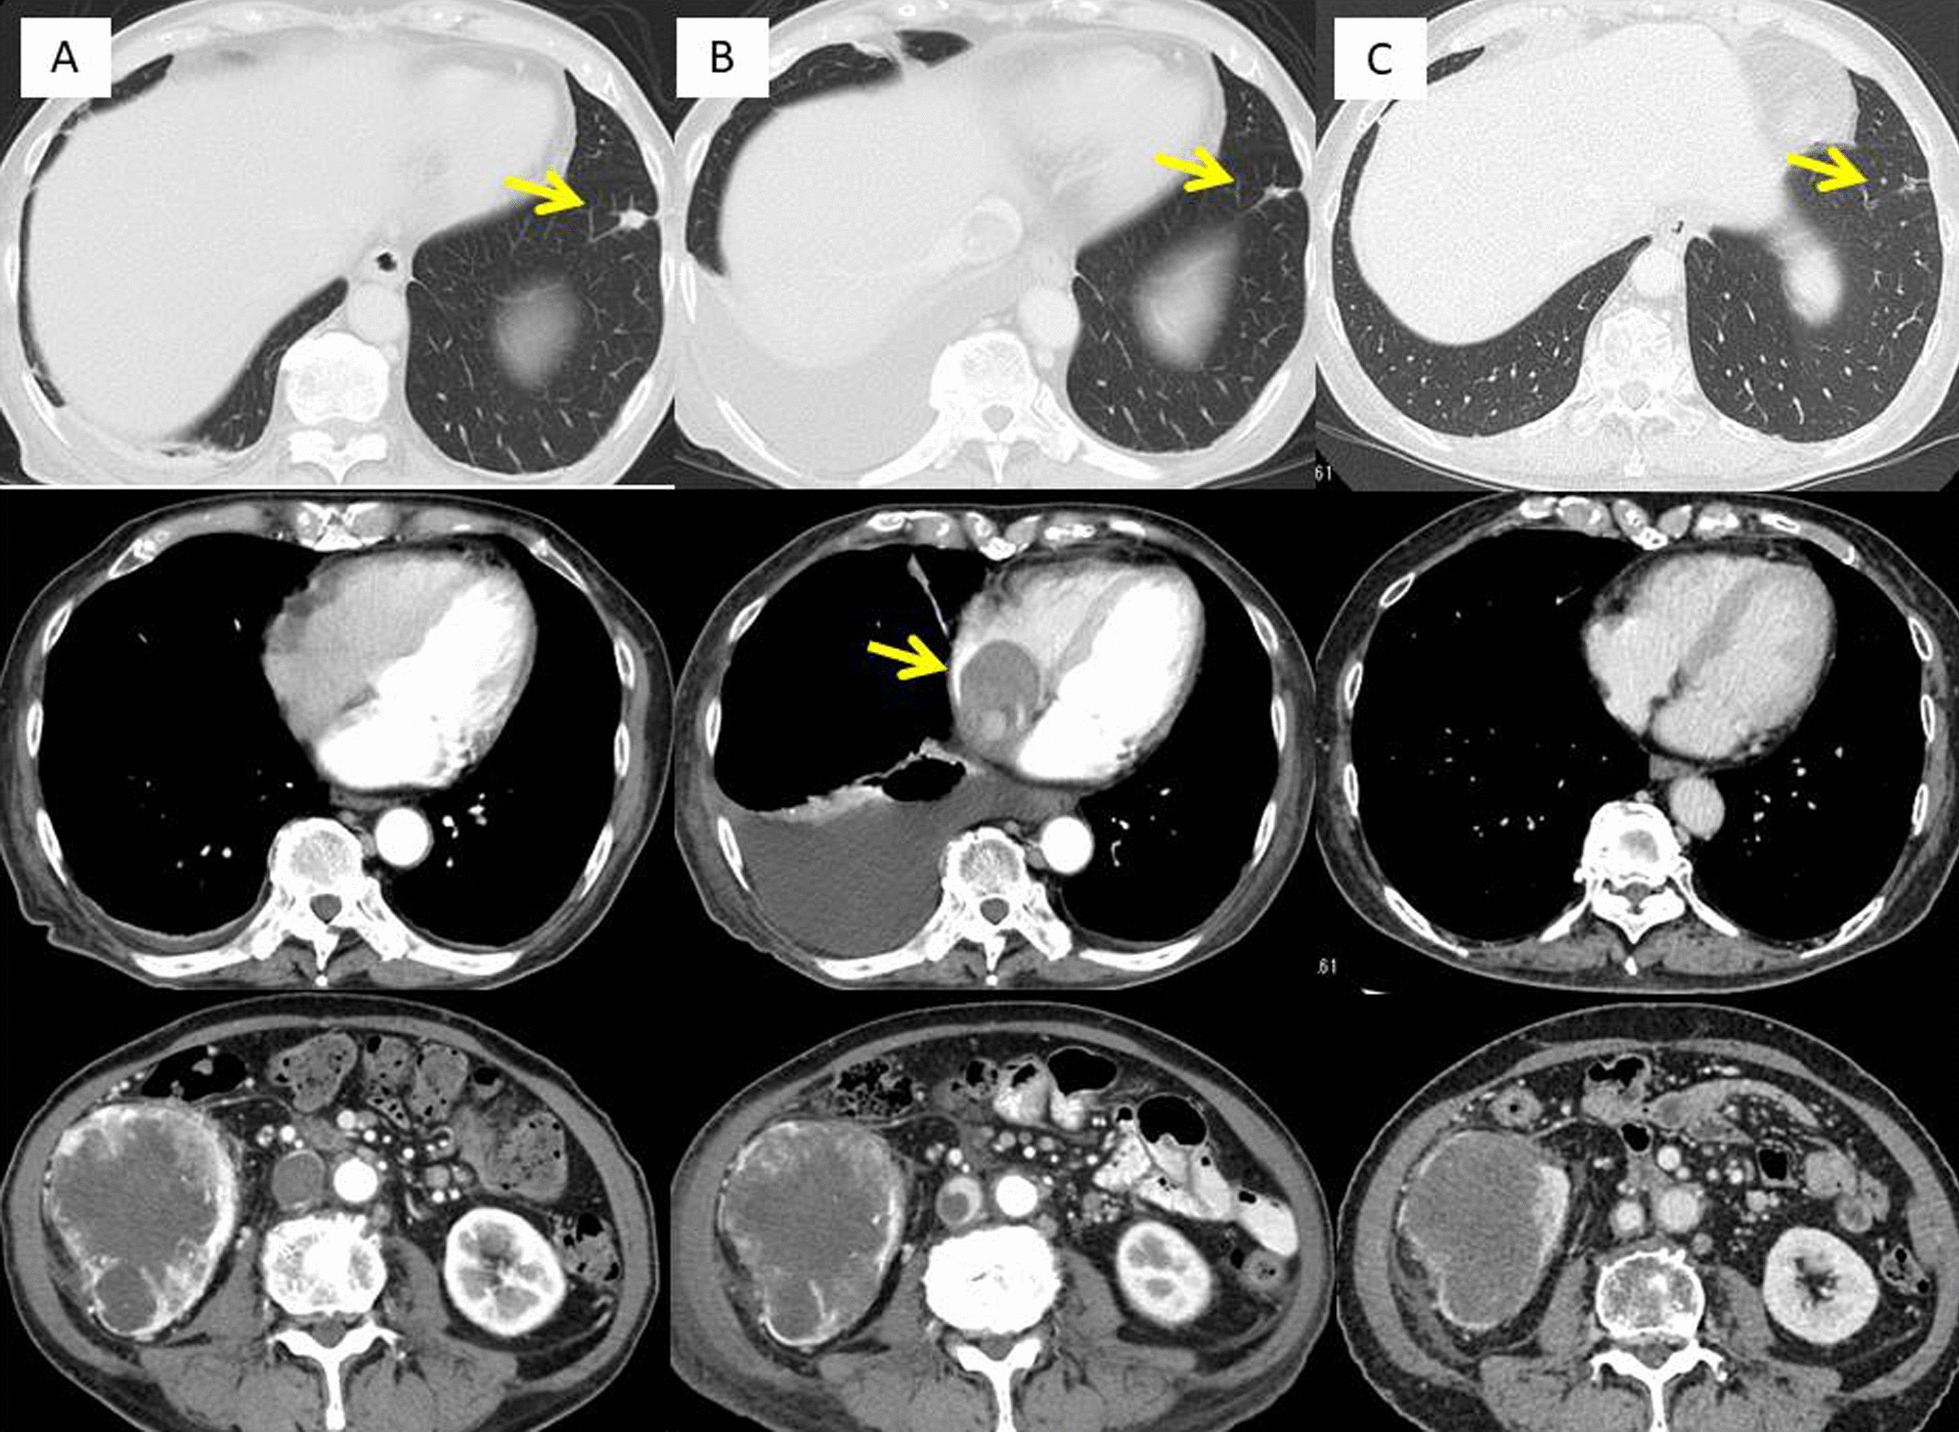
CT images showing lung metastasis and the primary tumor and tumor thrombus in the right atrium. a Before treatment. b After 2 cycles of nivolumab and ipilimumab. c Before surgery
